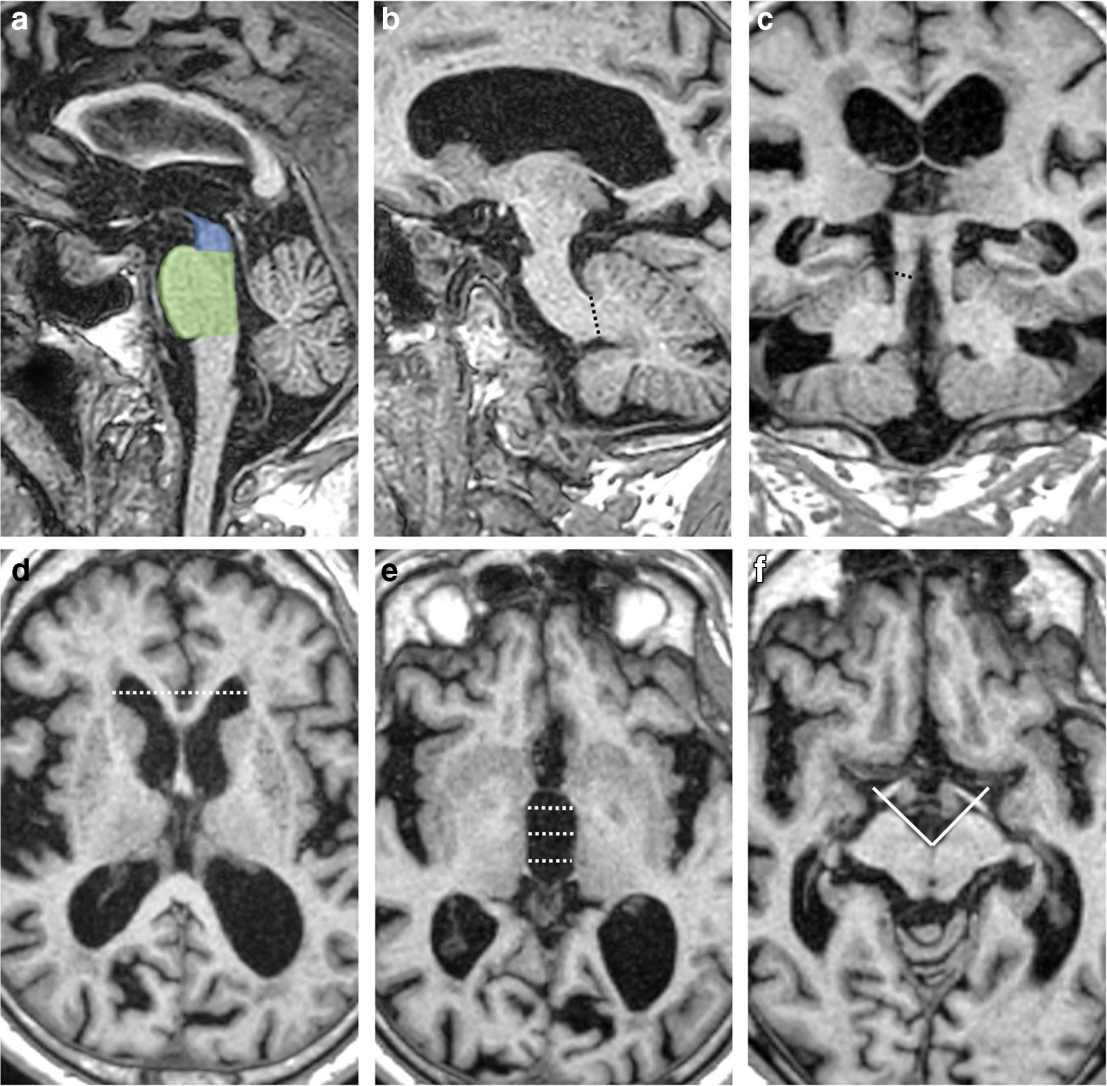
Sagittal (a–c) and axial (d–e) T1-weighted volumetric MR images of an iNPH patient showing sections on which MRPI and MRPI 2.0 measurements were performed. Midbrain and pons areas (a), middle (b), and superior (c) cerebellar peduncles thickness, frontal horn distance (d), and 3rd ventricle width (e) are depicted. Axial T1-weighted section on which interpeduncular angle was measured (f) is also shown
